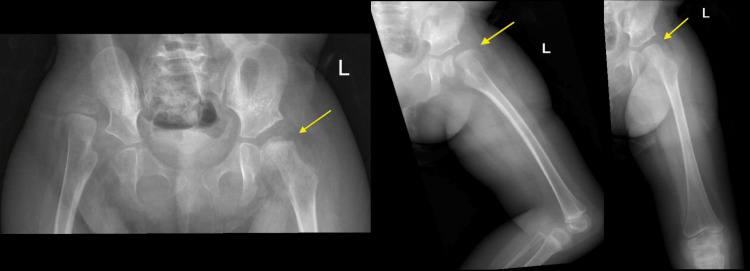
The X-ray of the patient's left femur at the time of injury
